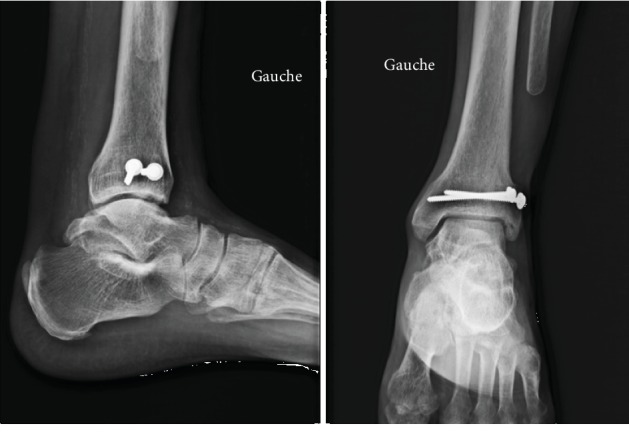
5-year postoperative radiography.
